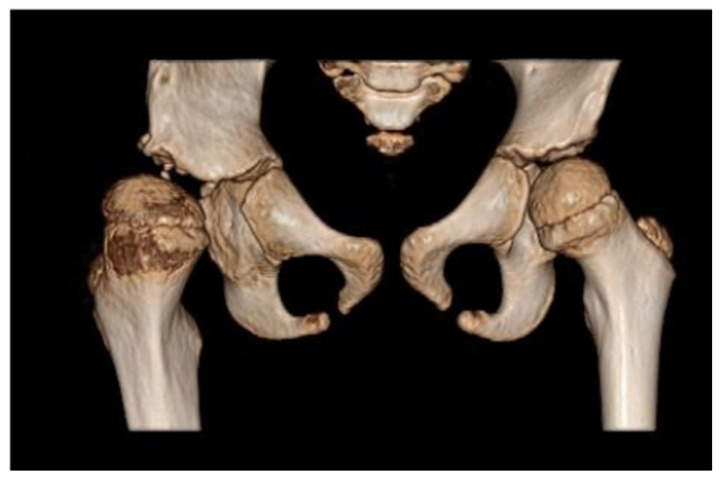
Computed tomography (CT) of the pelvis—3D scan carried out at 5 years of age. The relative tilt angle of the femoral head is about 47°; the angle of internal rotation is about 25°. Knee set in internal rotation. Femoral head in anterolateral subluxation. Joint space significantly widened. Thickening of soft tissues around the hip joint; exudate cannot be excluded. Differentiation reveals postoperative changes and inflammation. The acetabulum is slightly distorted in the area of the roof and flattened with irregular contours, marginal sclerotization, and small defects. A ~4 mm calcification or ossification can be seen in the soft tissues in front of the anterior part of the acetabular roof and below the posterior edge of the roof. The femoral head is flattened. There is marked thinning of the bone structure of the femoral head and neck.
